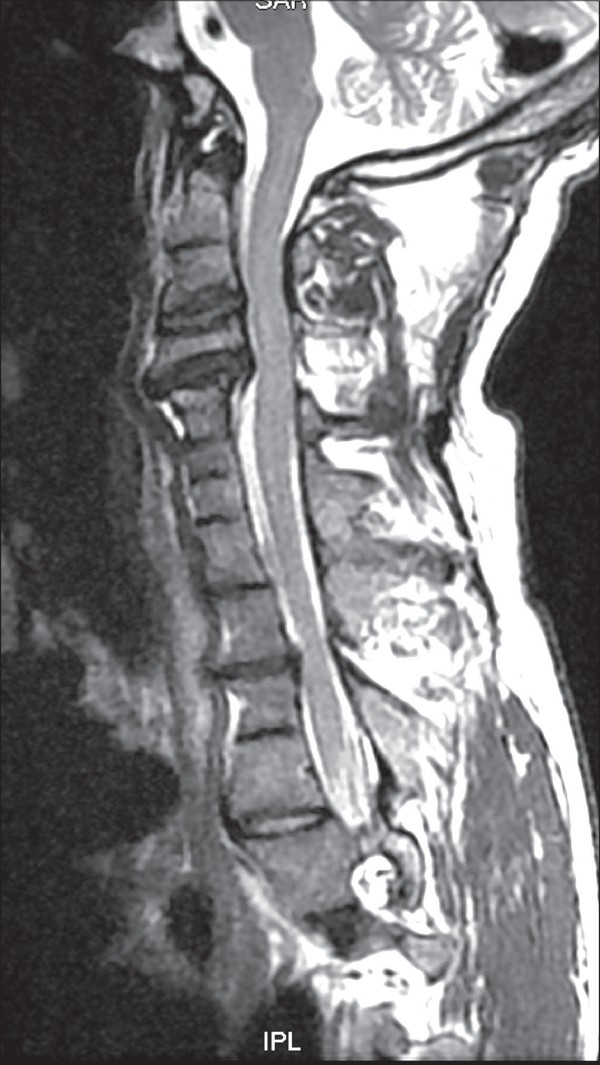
T2W sagittal MRI of the cervical spine shows atlantooccipital fusion and fusion of the C4-C6 vertebrae. The C2-C3 and C3-C4 intervertebral discs show posterior bulges
